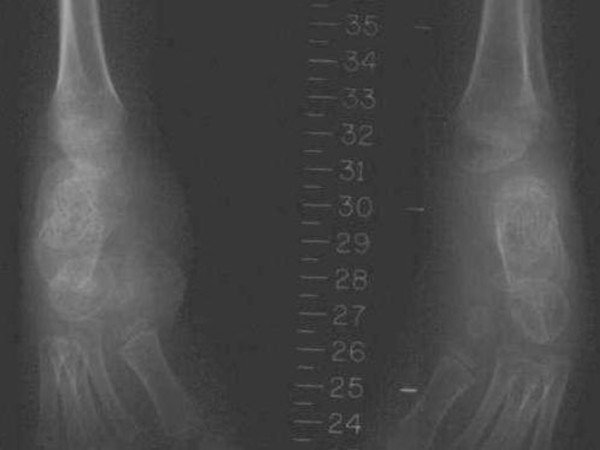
Anteroposterior radiograph of the feet showed complete resorption of the tarsal bones ended up with subsequent fusion of the melted bones and complete ankylosis with severe osteolysis.
